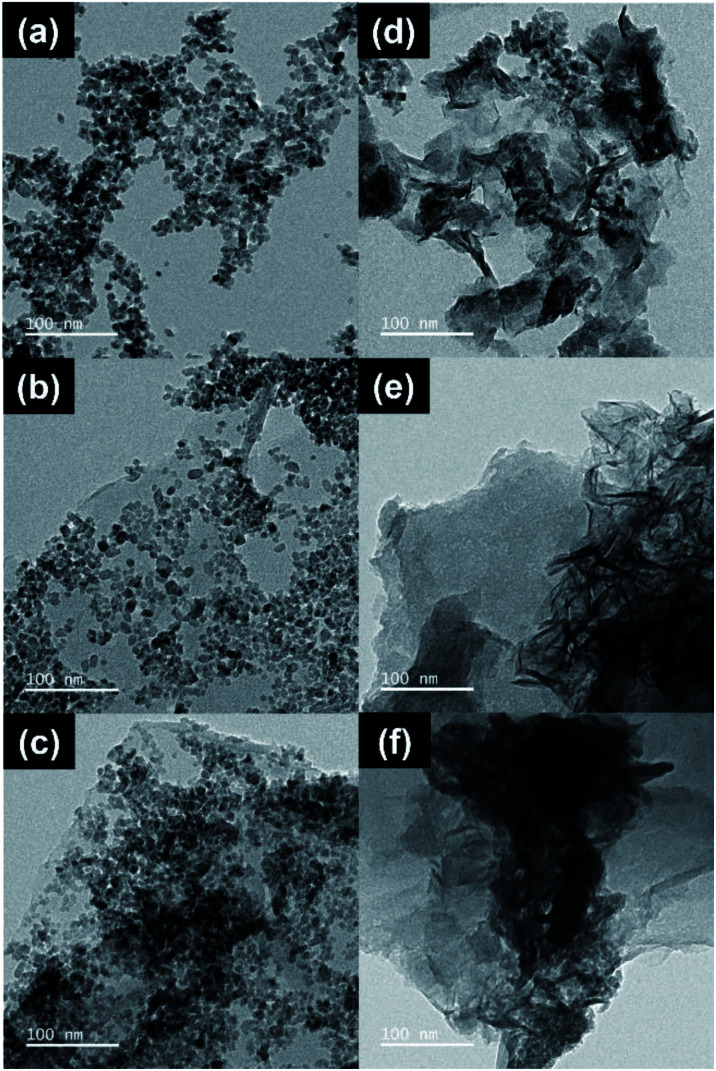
HR-TEM images showing CT (a), TG (b), CTG (c), CTN (d), TGN (e), and CTGN (f).
